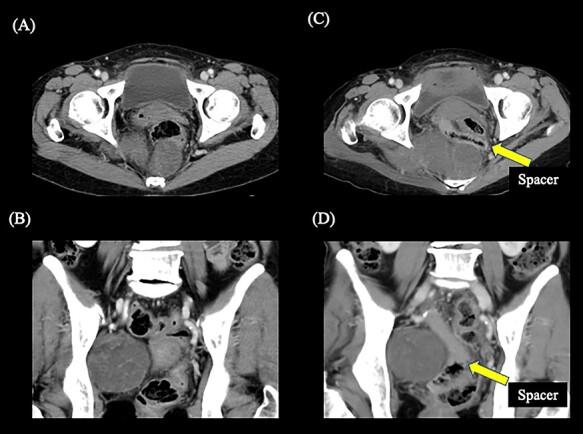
Contrast-enhanced computed tomography showing the positional relationship between the tumor and the rectum. (A) Axial image before spacer placement. (B) Coronal image before spacer placement. (C) Axial image after spacer placement. (D) Coronal image after spacer placement. The yellow arrow shows the spacer. The gas is formed within the spacer due to the process of hydrolysis after placement of the spacer.
